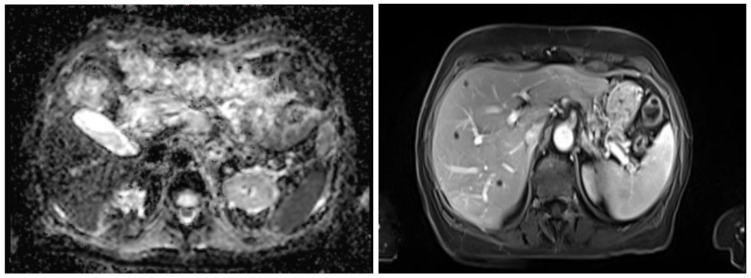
Example of a misclassified T1/T2 MRI image affected by noise or low contrast. (Left): Noisy image. (Right): Clear image (it is not the same image as the left one).
